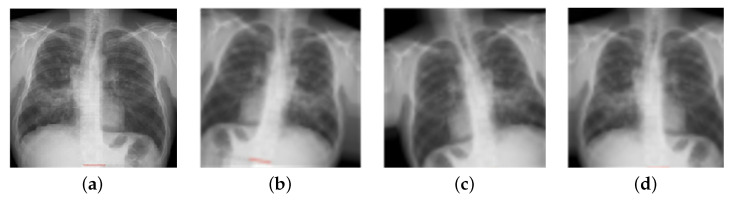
Images of chest X-rays after using random data augmentation techniques: (a) original, (b) after clockwise rotation by 10 degrees, (c) after anti-clockwise rotation by 10 degrees, and (d) after 10% translation.
